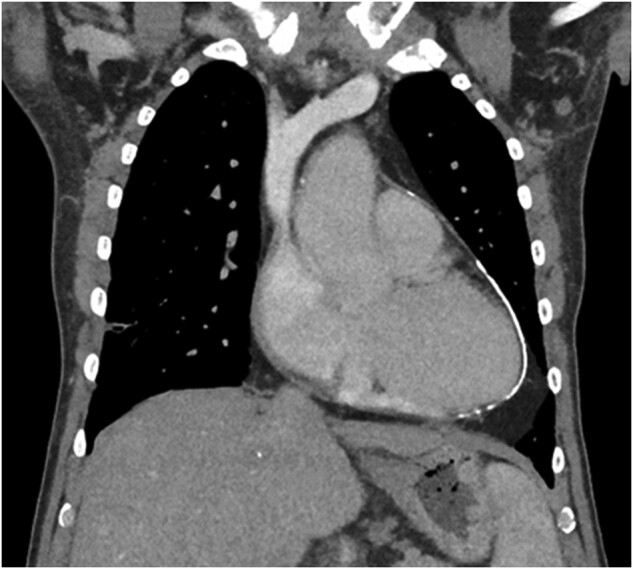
Coronal view of computed tomography thorax, demonstrating pericardial calcification.
